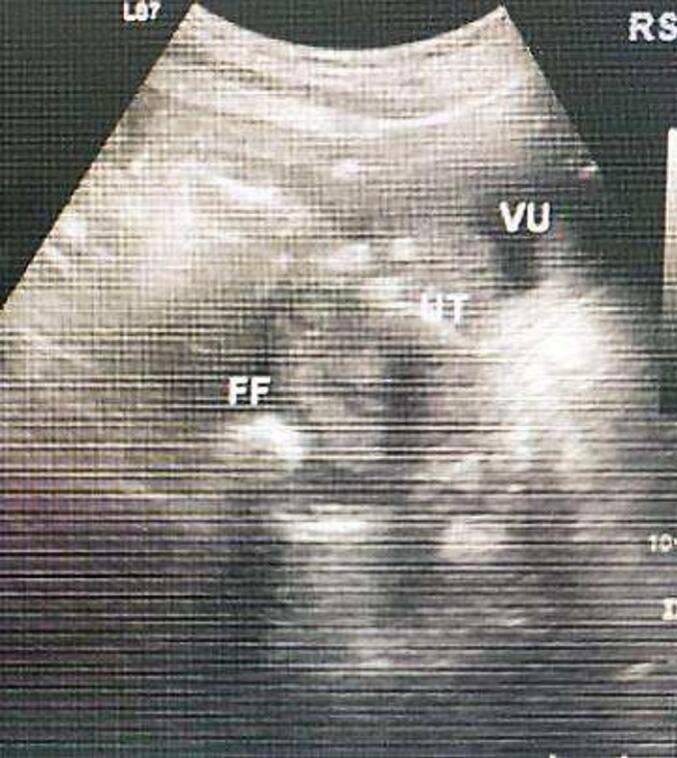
Free fluid in abdominal cavity found in abdominal ultrasound. FF: free fluid; UT: uterus; VU: vesical urinaria.
